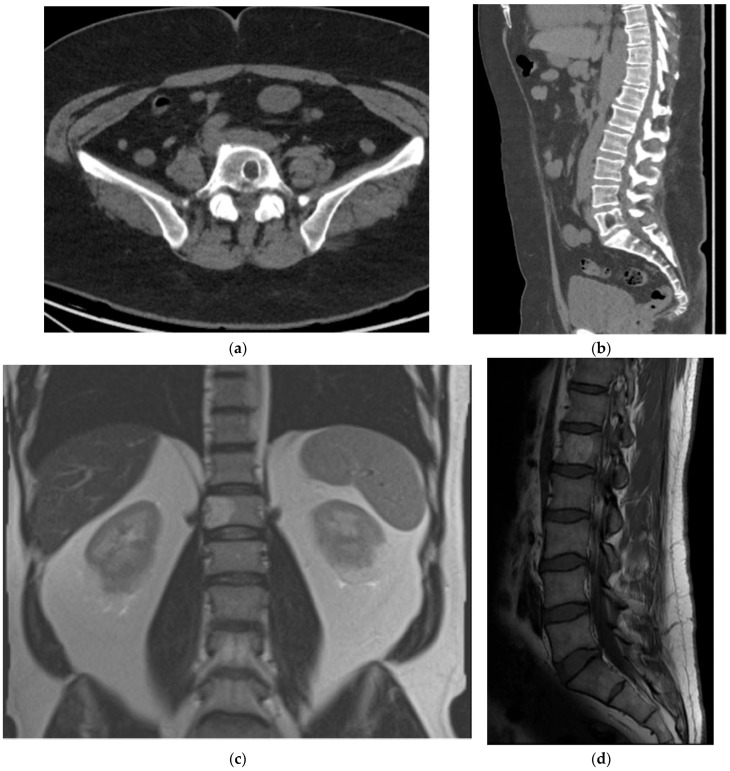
Lipoma. (a) Axial CT soft tissue window and (b) sagittal CT soft tissue window demonstrate a well-circumscribed, non-expansile, fat-attenuating lesion within the L5 vertebral body. The lesion is consistent with intraosseous lipoma. (c) Coronal T2 MR image shows a non-expansile intraosseous lesion confined within the vertebral body, with similar signal intensity to fat, in keeping with intraosseous lipoma. (d) Sagittal T1 MR (fast spin echo sequence) demonstrates the same intraosseous lipoma as in Figure 22c.
